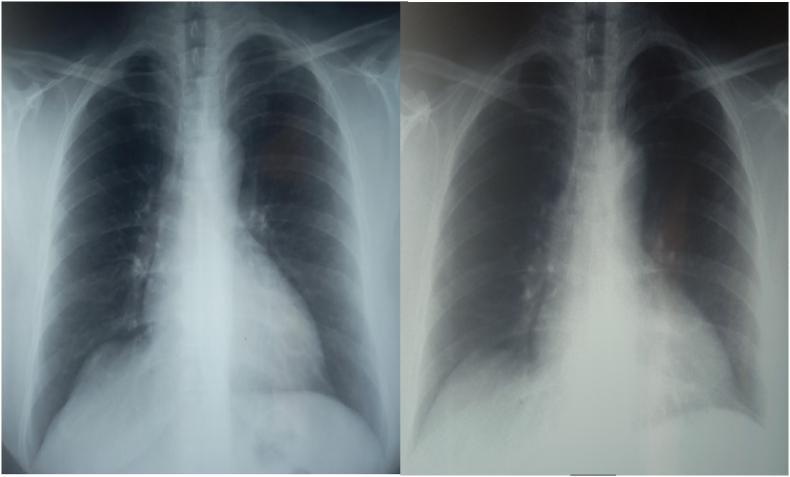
Chest X-ray: improvement of radiological lesions over two years.
